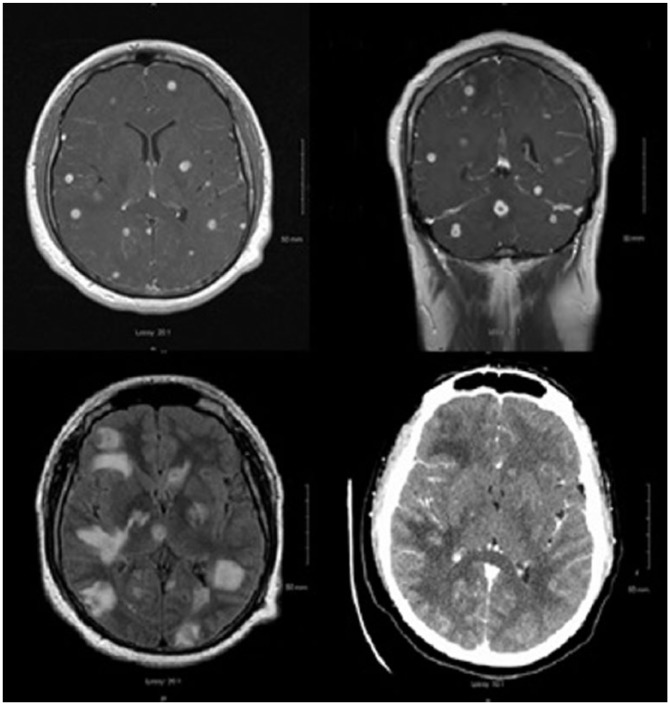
Magnetic resonance imaging (MRI) with numerous ring-enhancing lesions
